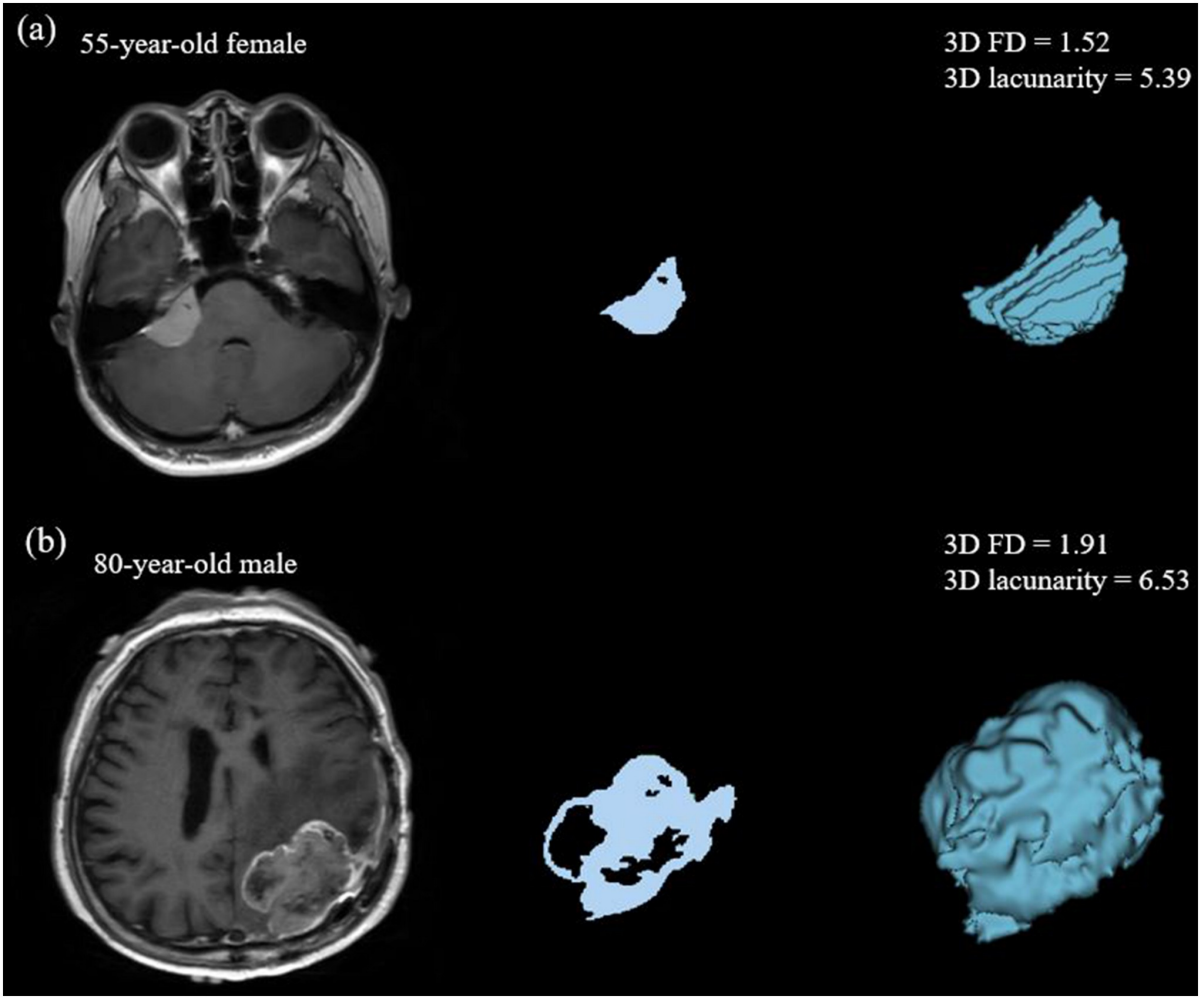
Representative cases of fractal parameters in grade 2 meningiomas according to TERTp mutation status. (a) Images of a 55-year-old female with TERTp wildtype grade 2 meningioma reveals an enhancing tumor in the right cerebellopontine angle on postcontrast T1-weighted image with a major axis of 2.9 cm. The 3D FD and 3D lacunarity were 1.52 and 5.39, respectively. (b) Images of an 80-year-old male with TERTp mutant grade 2 meningioma reveals an enhancing tumor in the left parietal convexity on T1C with a major axis of 5.3 cm. The 3D FD and 3D lacunarity were 1.91 and 6.53, respectively. FD = Fractal dimension, TERTp = telomerase reverse transcriptase promoter, T1C = Postcontrast T1 weighted image.
